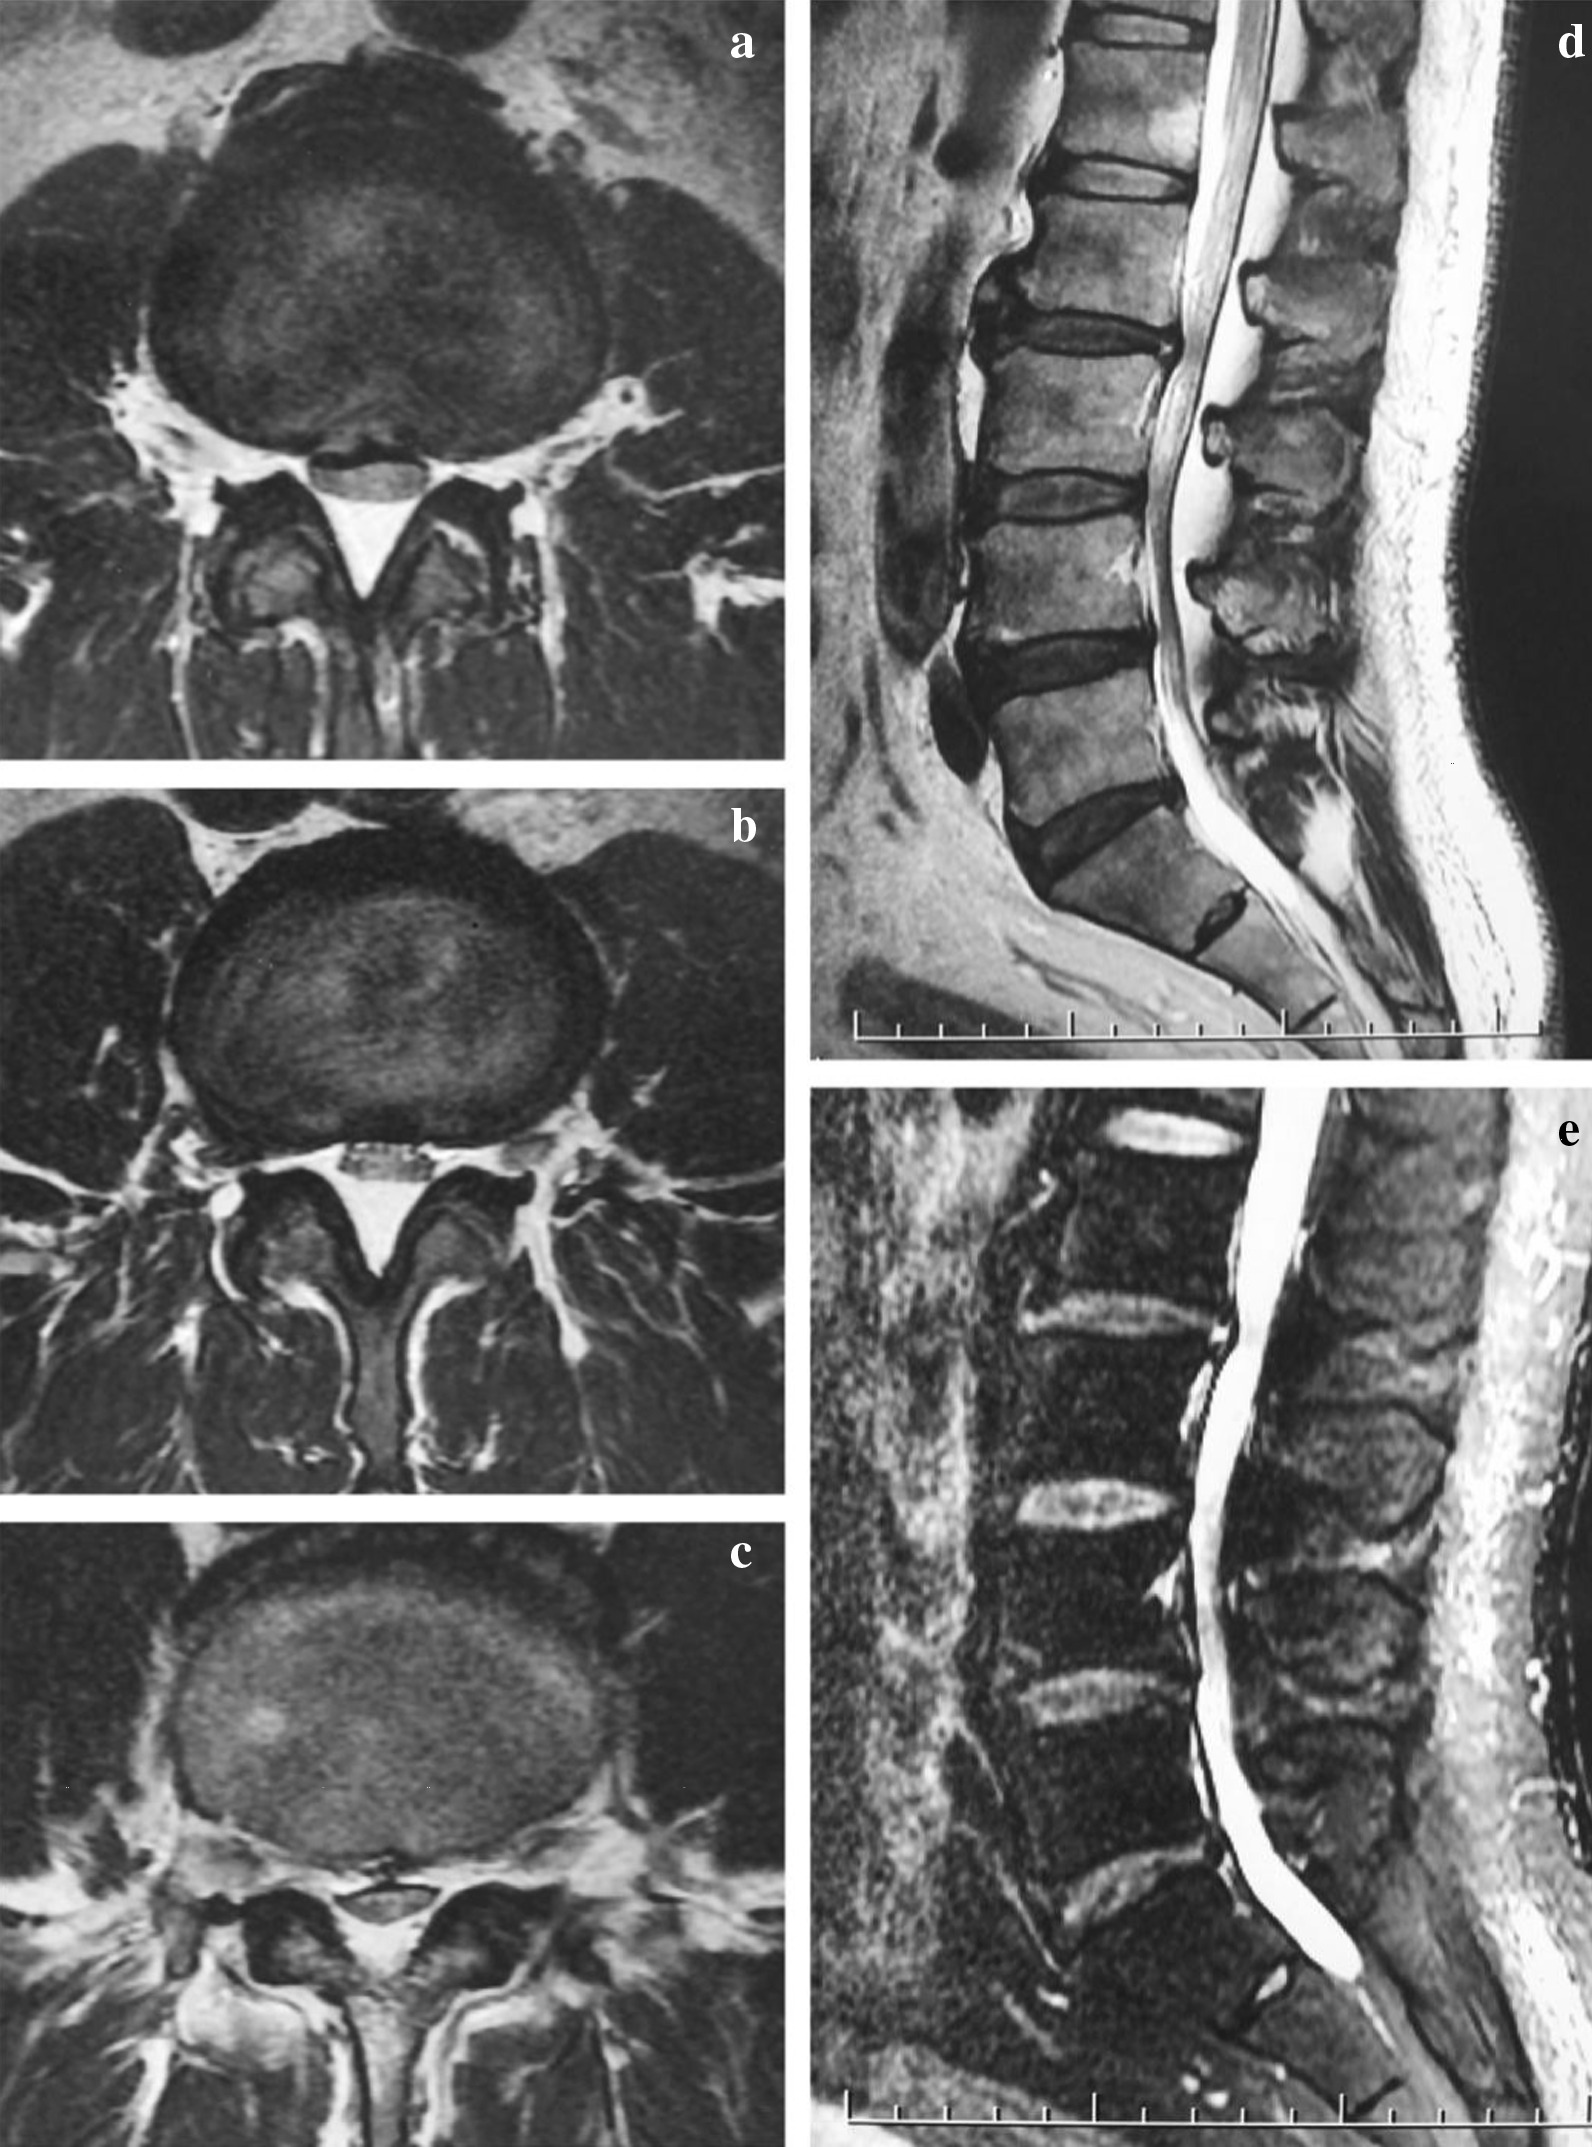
Preoperative MRI. T2-weighted axial images show classic “Y-sign” thecal compression secondary to epidural lipomatosis from L2-L5. a Axial image of L2/3. b Axial image of L3/4. c Axial image of L4/5. d T2-weighted sagittal image shows epidural lipomatosis as short homogeneous signal, compression and ventral shift spinal cord. e Fat suppression image shows suppression of adipose tissue
